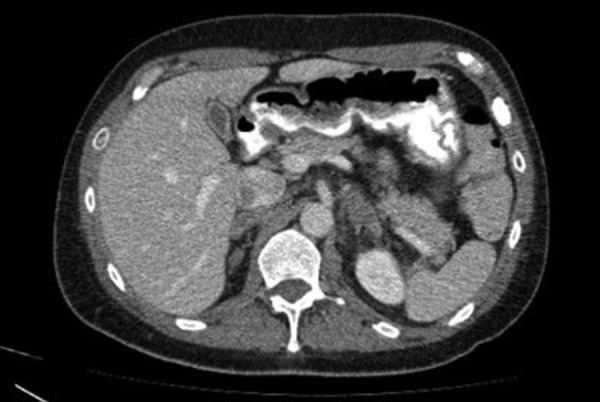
Abdominal computed tomography (CT) revealing gallbladder edema without gallstones and pericholecystic fluid.
